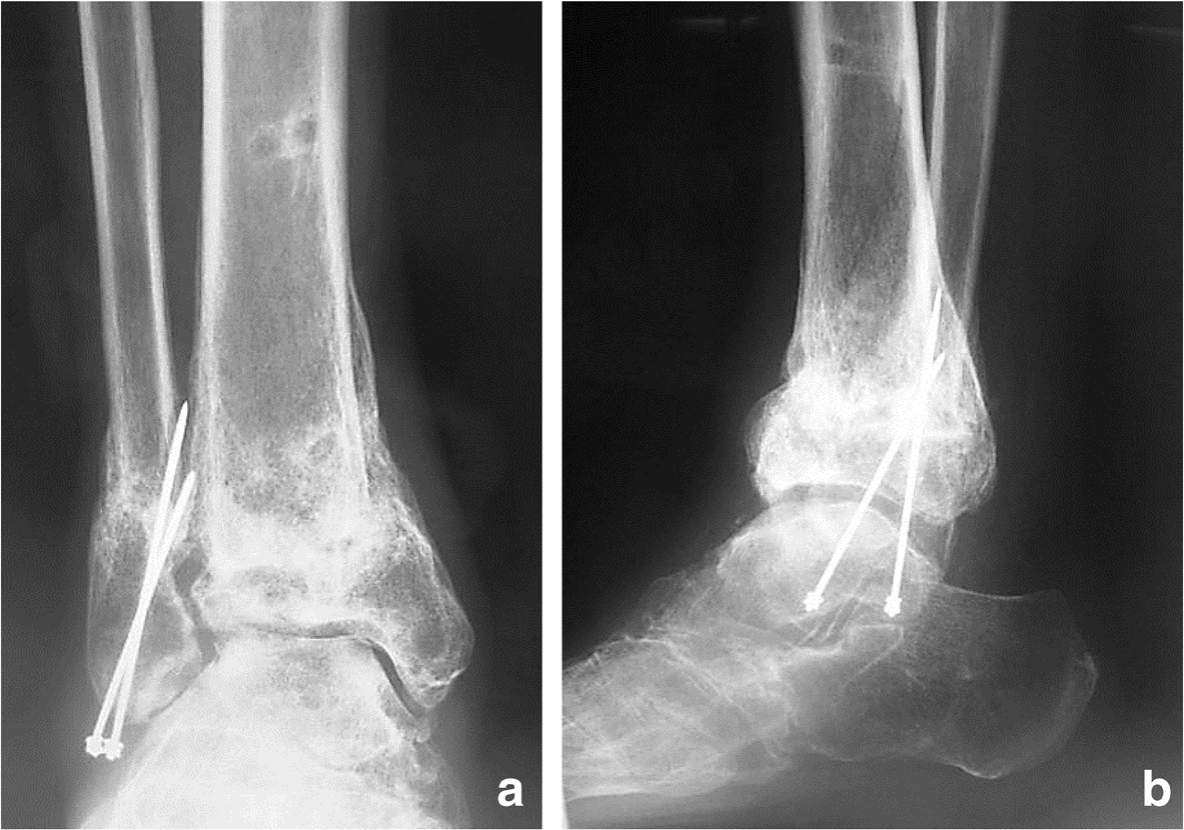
Postoperative radiographs. (a, b) Radiograph after removal of the external fixator, showing bone union.
